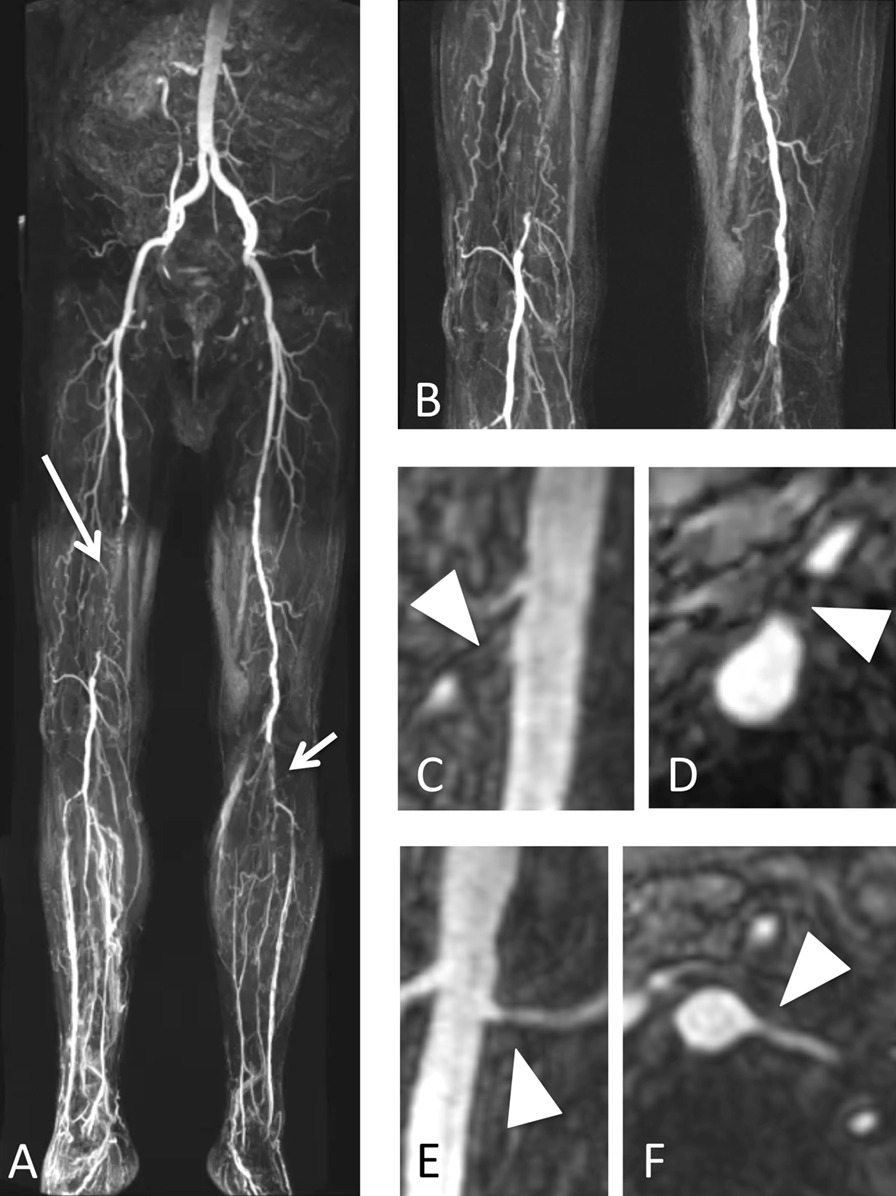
Run-off MR angiography in a 74-year old male patient with known Stage IV peripheral artery disease. Subtracted images post contrast show occlusion of the distal portion of the right superficial femoral artery (long arrow in a, magnified in b) and of the left popliteal artery segment P3 (short arrow in a, magnified in b). T1-weighted post-contrast images of the visceral arteries demonstrate an occlusion of the superior mesenteric artery in the sagittal and oblique transverse plane (arrowhead in c and d) and a mild stenosis of the left renal artery, which was classified as grade 2, in the coronal and oblique transverse plane (arrowhead in e and f)
